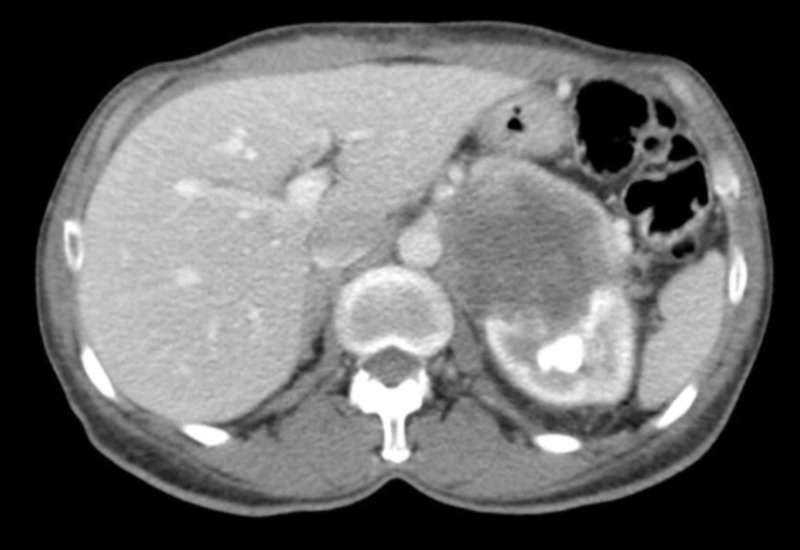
Contrast-enhanced CT scan of the abdomen showing progression of the left kidney cancer.
